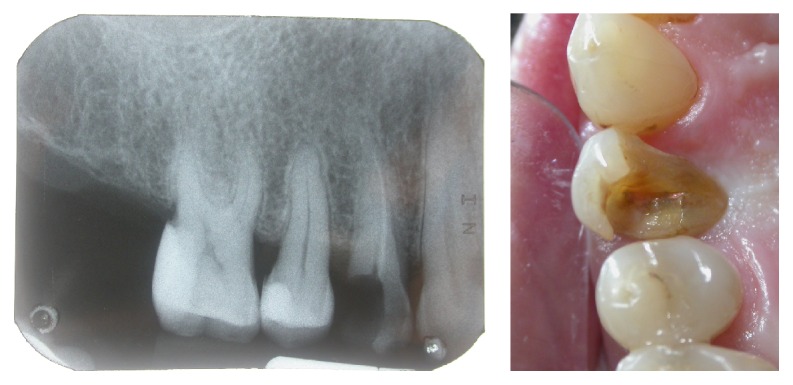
Case 2: clinical and radiographic evaluation before the extraction.
